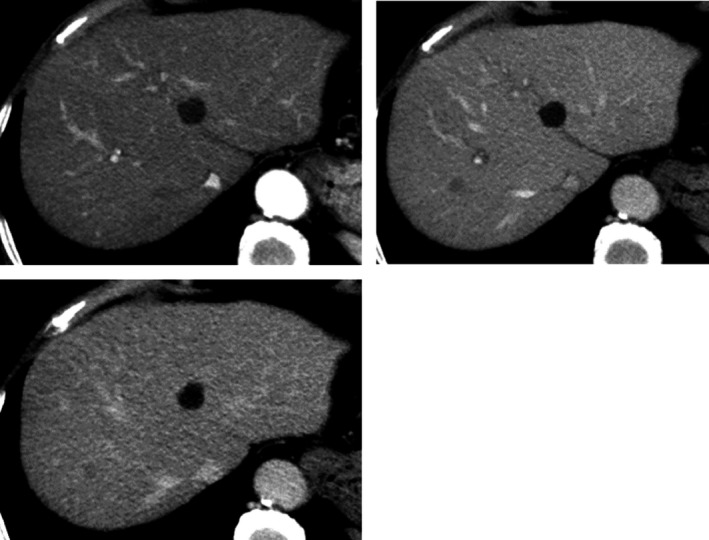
On abdominal computed tomography (CT) scan, a mass, 10 mm in diameter, was demonstrated, which was slightly enhanced in the early arterial phase and subsequently washed out in the late phase after contrast material injection, but was not consistent with a HCC. Other organs, including regional or para‐aortic lymph nodes, showed no abnormal findings
